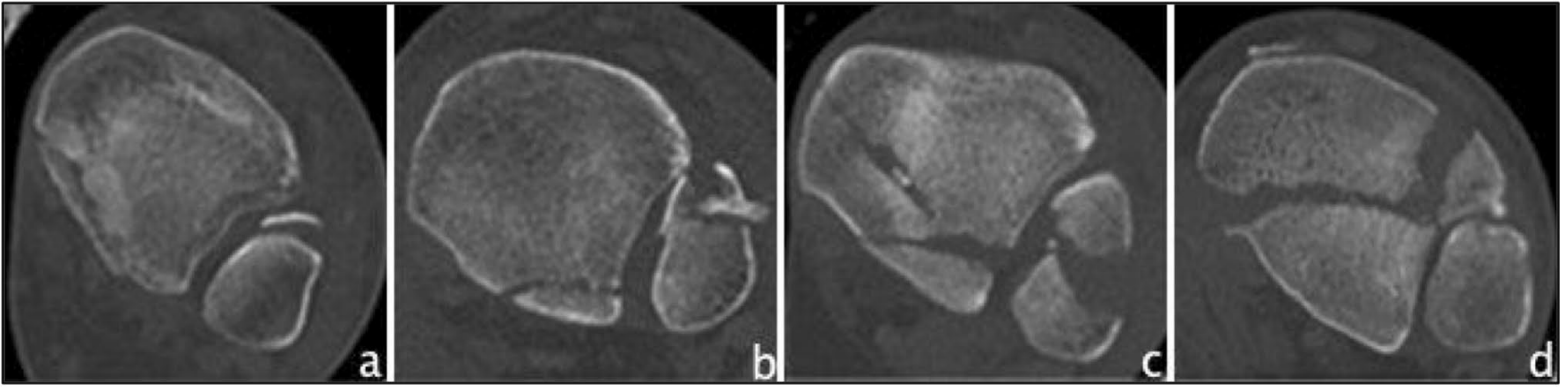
Overview of the Bartoníček/Rammelt classification based on CT images (axial views). a Bartoníček type 1 b Bartoníček type 2 c Bartoníček type 3 d Bartoníček type 4
